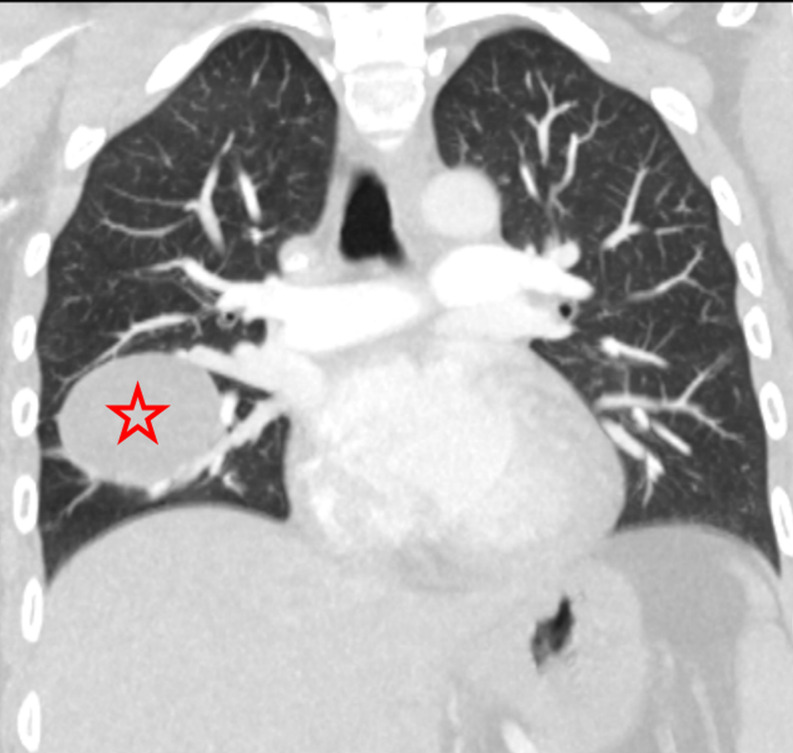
Coronal section of chest computed tomography (CT) with lung window in a 32-year-old female patient showing an intact hydatid cyst (asterisk).
